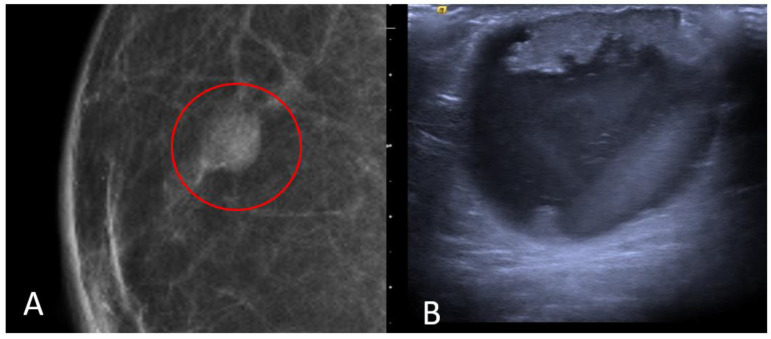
Invasive papillary carcinoma in a 59-year-old man with bloody nipple discharge. Magnification of mediolateral oblique view of the right breast (A) shows a focal high-density mass with well-circumscribed margins (red circle). Ultrasound (B) shows a complex cyst with mixed solid and cystic morphologic features.
